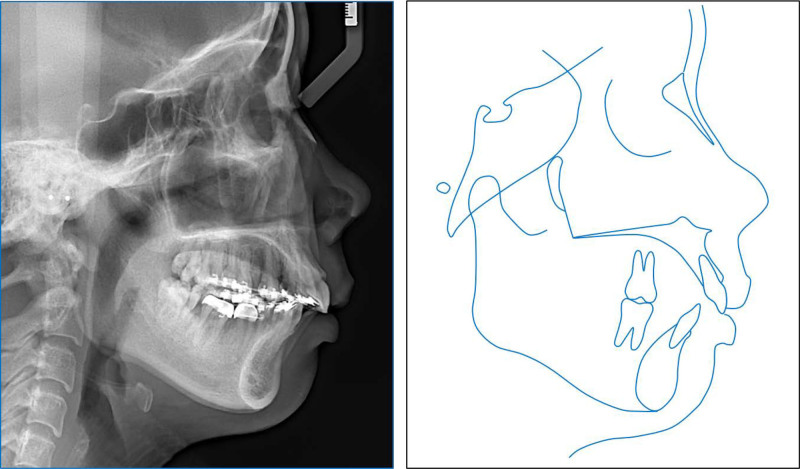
Presurgical cephalometric radiograph and tracing.
